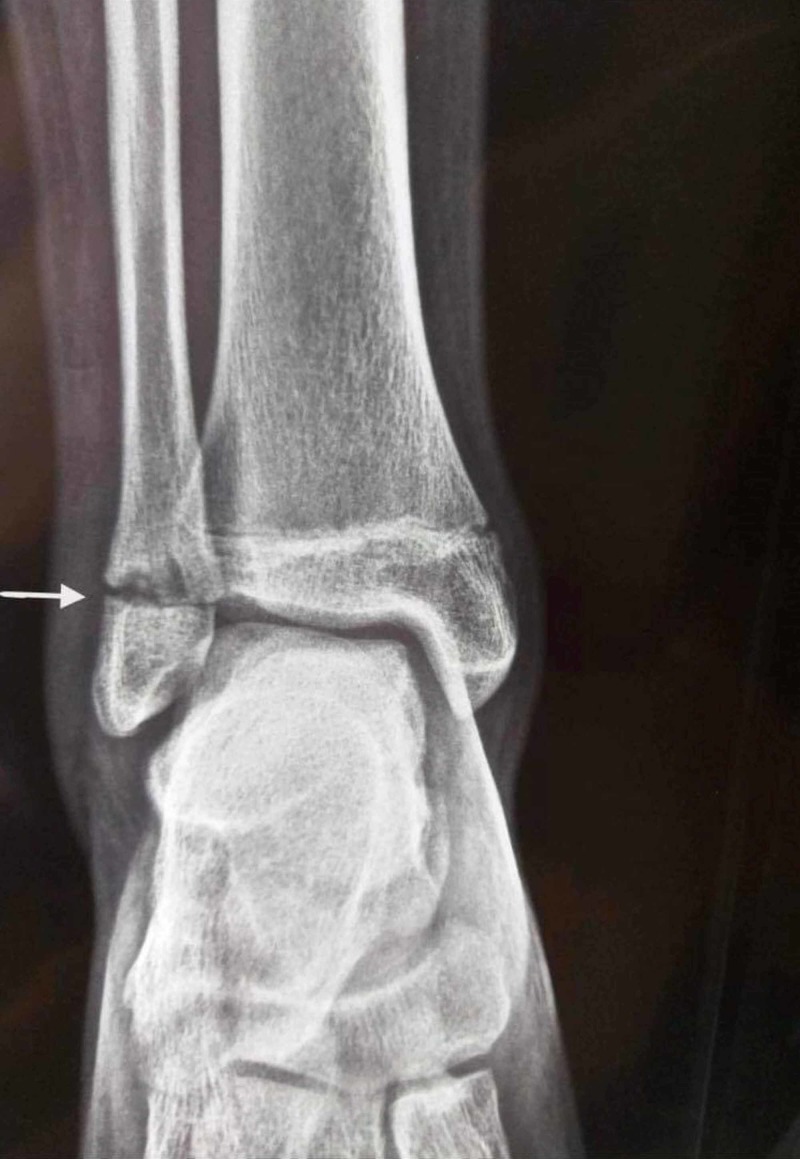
X-ray of the right ankle Osteolytic changes of the right fibula (white arrow)
